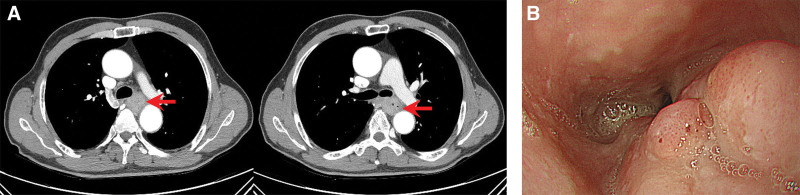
Computed tomography and esophagogastroduodenoscopy. (A) Computed tomography of the chest showed enhanced wall thickening and luminal narrowing of the mid-esophagus. (B) Esophagogastroduodenoscopy revealed an eccentric ulceroinfiltrative mass in the lower thoracic esophagus at 26 cm from the incisors.
